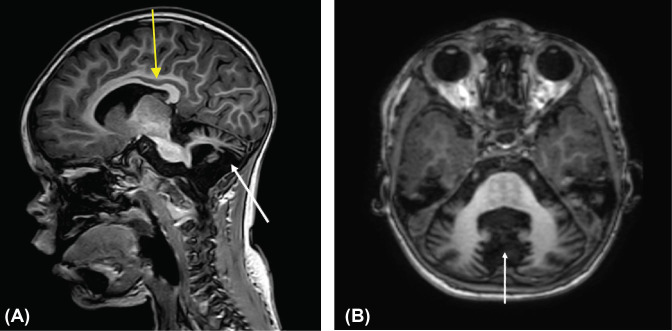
Twenty-one month old child with cerebellar hypoplasia. (A) Mid-sagittal T1-weighted image showing thinning of corpus callosum (yellow arrow) and decreased cerebellar volume (white arrow). (B) Axial T1-weighted image showing decreased volume of the cerebellar lobes (white arrow). With cerebellar hypoplasia, hemispheres are normal, but patients have small brain stems, particularly the pons and medulla. Other structural brain abnormalities may include thinning or absence of the corpus callosumaand communicating hydrocephalus.
